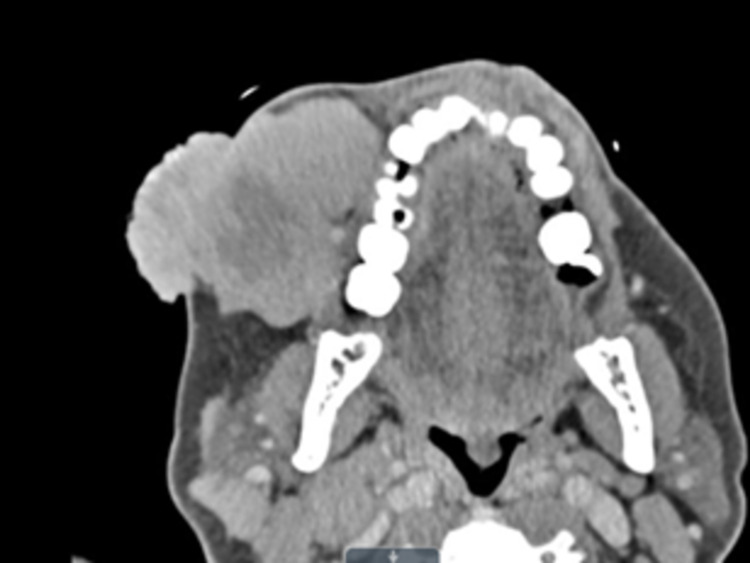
CT scan of the Merkel cell carcinoma, axial view
